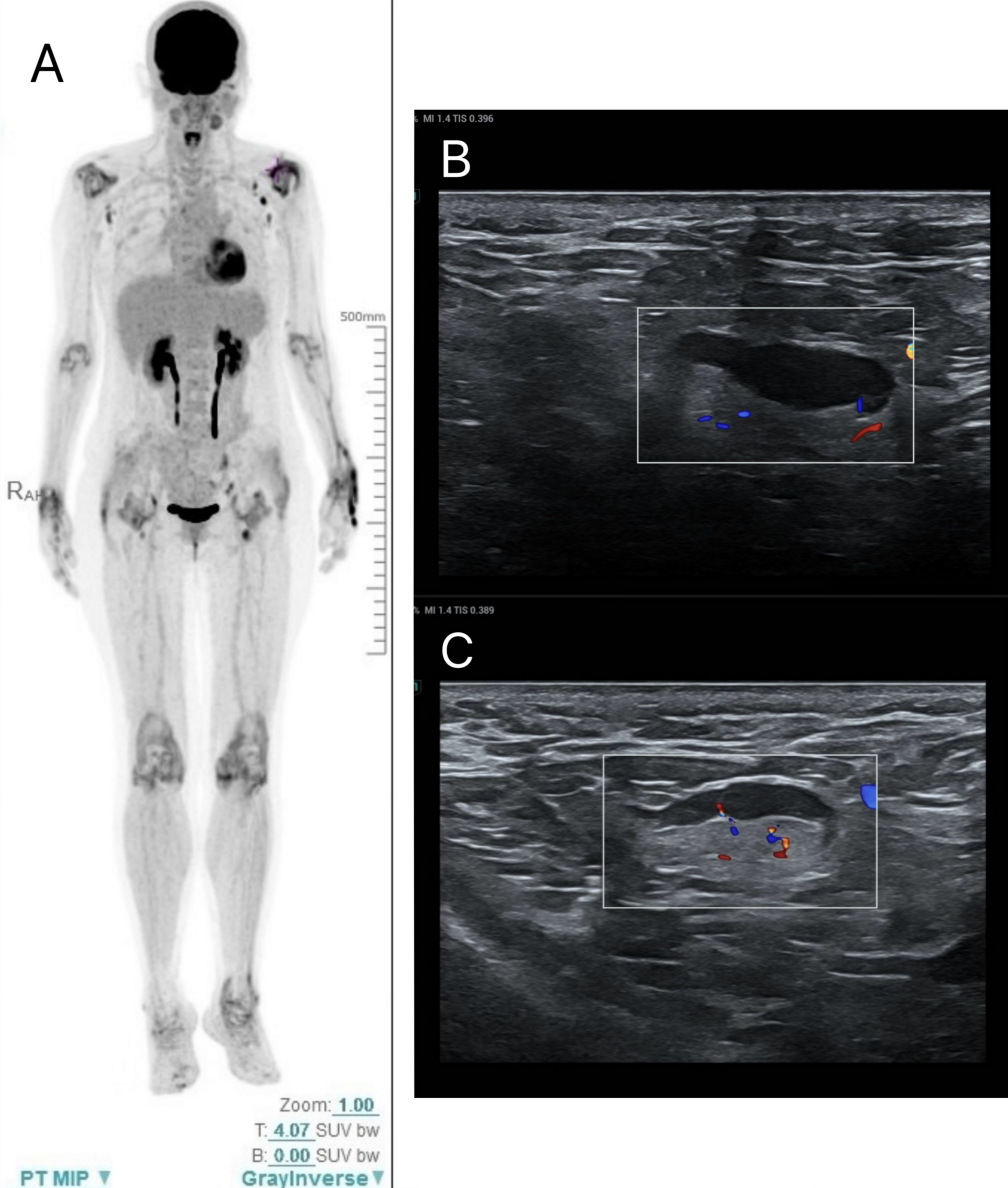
PET-CT showing diffusely increased metabolic activity in the periarticular soft tissues of the extremities and axillary lymph node ultrasound A: After fasting for over six hours and an intravenous injection of ¹⁸F-FDG, diffuse metabolic uptake is observed in the periarticular soft tissues of bilateral wrists, elbows, shoulders, hips, knees, and ankles (SUVmax ~5.5). B-C: Ultrasound demonstrates enlarged bilateral axillary lymph nodes.
